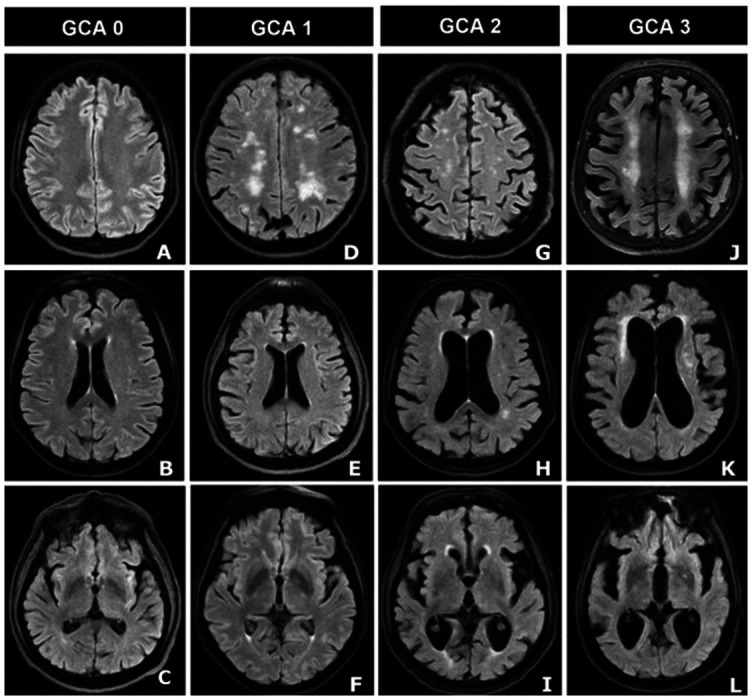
Axial FLAIR images of 4 different male patients, aged 66, 72, 86, and 82 years, with clinical Alzheimers’s disease manifestation showing global cortical atrophy and ventricular dilatation in different stages. Using the Pasquier scale with O’Donovan addition in the assessment of ventricular enlargement, the first column shows: (A) normal volume of the gyri and width of the sulci, (B) normal dilatation of lateral ventricles, and (C) normal dilatation of the third ventricle. The second column shows: (D) mild atrophy with a still normal volume of the gyri but some open sulci, (E) mild dilatation of lateral ventricles, (F) mild dilatation of the third ventricle. The third column shows: (G) moderate brain atrophy with a reduction of gyri volume, and enlargement of the sulci, (H) moderate dilatation of lateral ventricles, (I) moderate dilatation of the third ventricle. The fourth column shows: (J) severe atrophy with severely reduced gyri, and enlarged sulci, (K) severe dilatation of lateral ventricles, (L) severe dilatation of the third ventricle. FLAIR: Three-dimensional T2-weighted fluid-attenuated inversion-recovery imaging.
